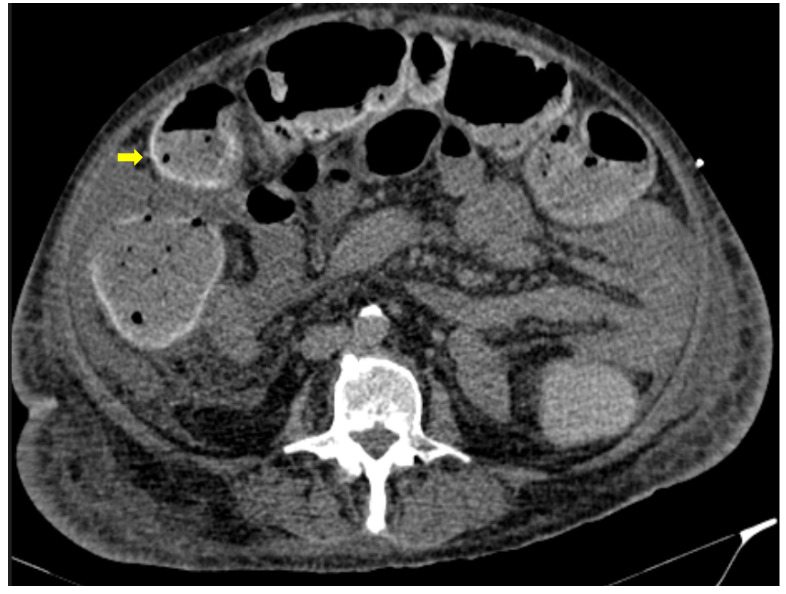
59-year-old female patient with severe COVID-19 pneumonia hospitalized in the ICU. CT demonstrating distended large bowel with hyperenhanced walls and free abdominal fluid (shock bowel)—yellow arrow. Note the concomitant subcutaneous edema.
